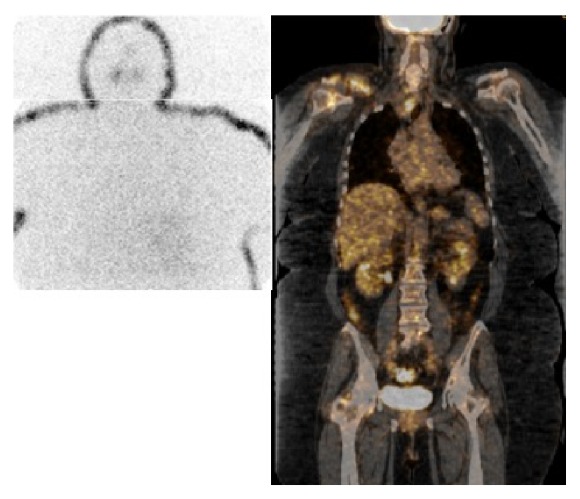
Left: posttherapy 131-iodine WBS shows no evidence of functional thyroid tissue. Right: coronal-fused images of 18F-FDG PET/CT study performed after posttherapy WBS show the FDG avid mediastinal and right shoulder masses.
